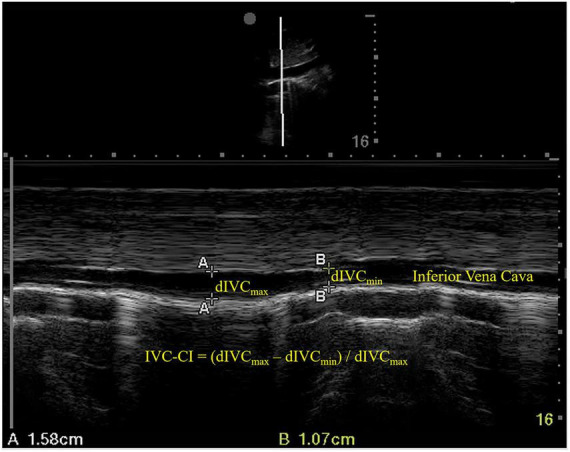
Ultrasound measurements of the inferior vena cava. dIVC_max_, maximum inferior vena cava diameter; dIVC_min_, minimum inferior vena cava diameter; IVC-CI, collapsibility index of inferior vena cava.
